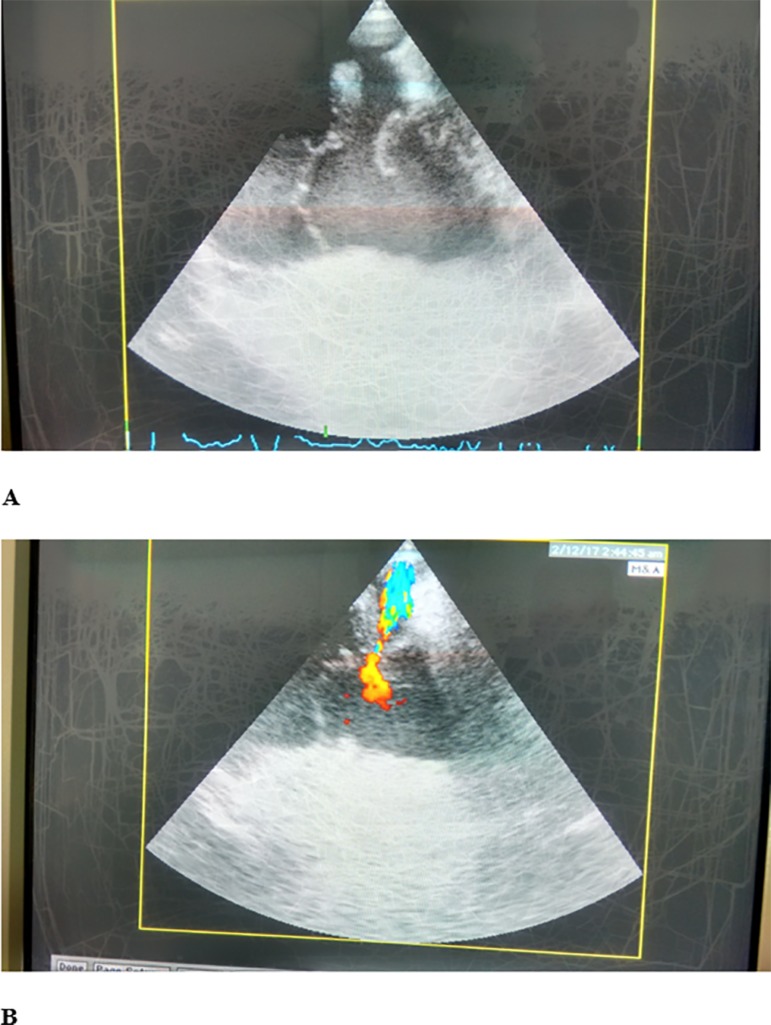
Snapshots during transesophageal echocardiography examination (transverse
